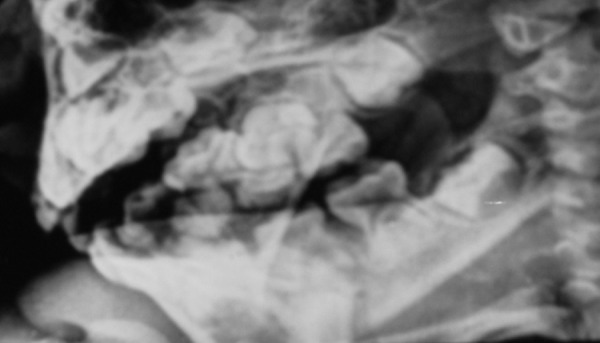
Lateral oblique X-ray of undisplaced body of mandible
